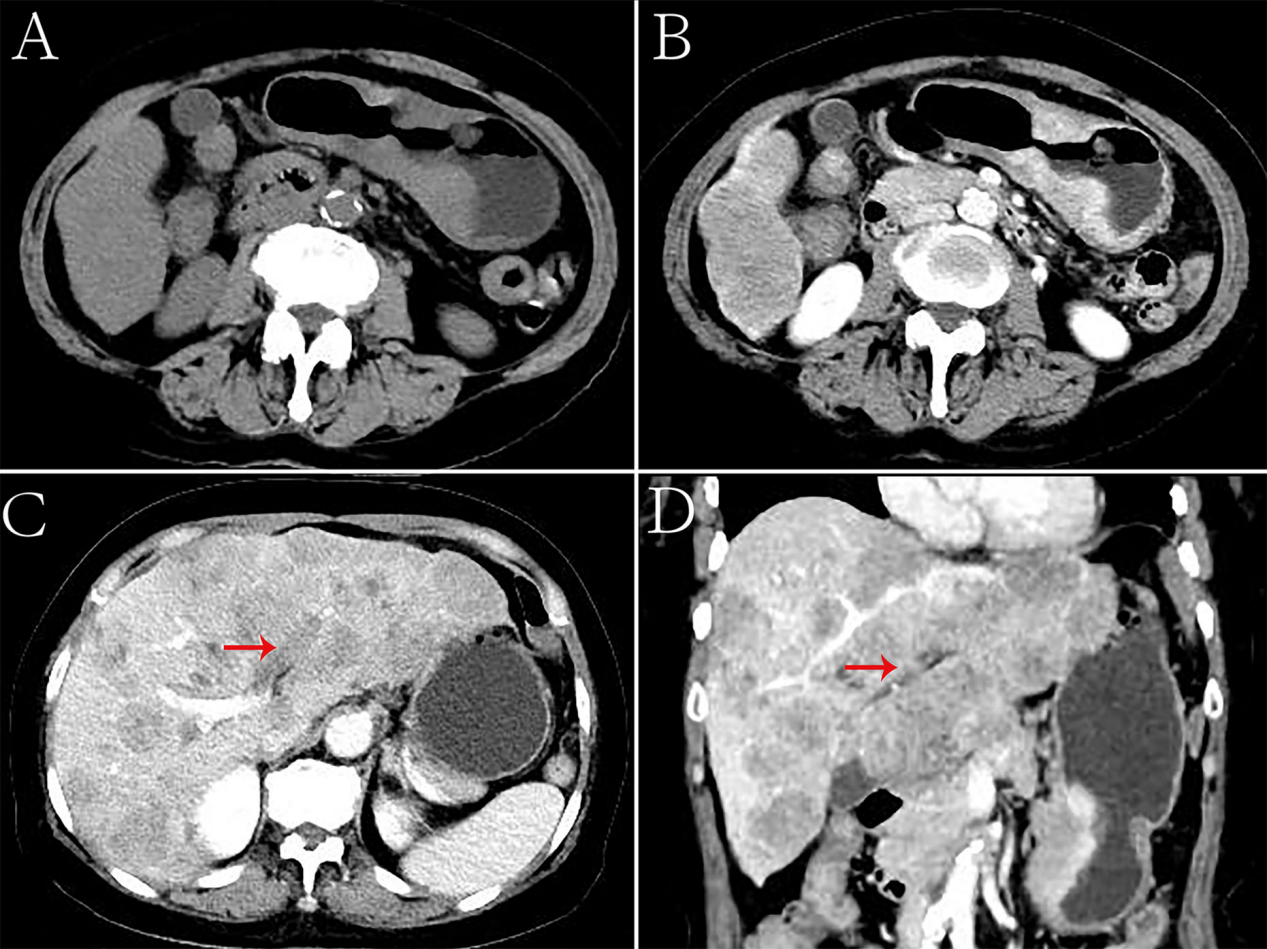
Hepatoid adenocarcinoma of the stomach with embolization of the left branch of the portal vein in a 70-year-old woman. (A) Unenhanced computed tomography (CT) image of the stomach showing an intraluminal mass of inhomogeneous attenuation, with an undercooling boundary, in the cardia and fundus. The CT attenuation was approximately 41 Hounsfield units (HU). Multiple nodular low-density metastases of different sizes were found in the liver. (B–D) Contrast-enhanced CT image showing obvious inhomogeneous enhancement of the mass. The thickest diameter of the mass was 3.3 cm. The Borrmann classification was type III. The arterial and venous phases showed a similar CT attenuation (approximately 91 HU). Intrahepatic metastases showed mild to moderate enhancement on enhanced CT. The left branch of the portal vein had no obvious changes, and low-density emboli could be seen in the lumen. (C) Portal phase of the contrast-enhanced image. (D) Portal phase of the contrast-enhanced coronal image.
